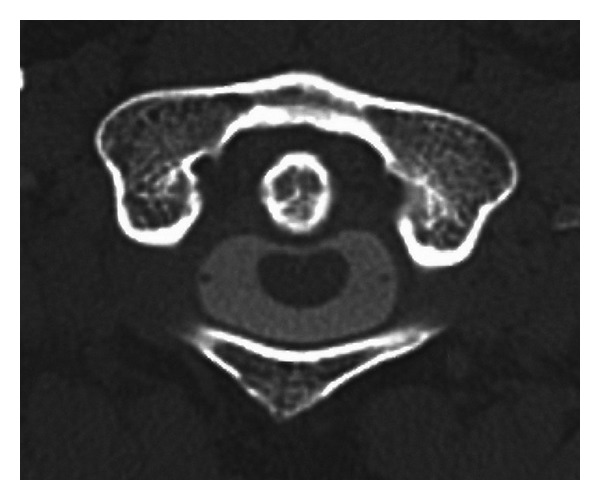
CT images after myelography. An increased ADI was apparent without evidence of fracture at the attachment site of the transverse ligament.
